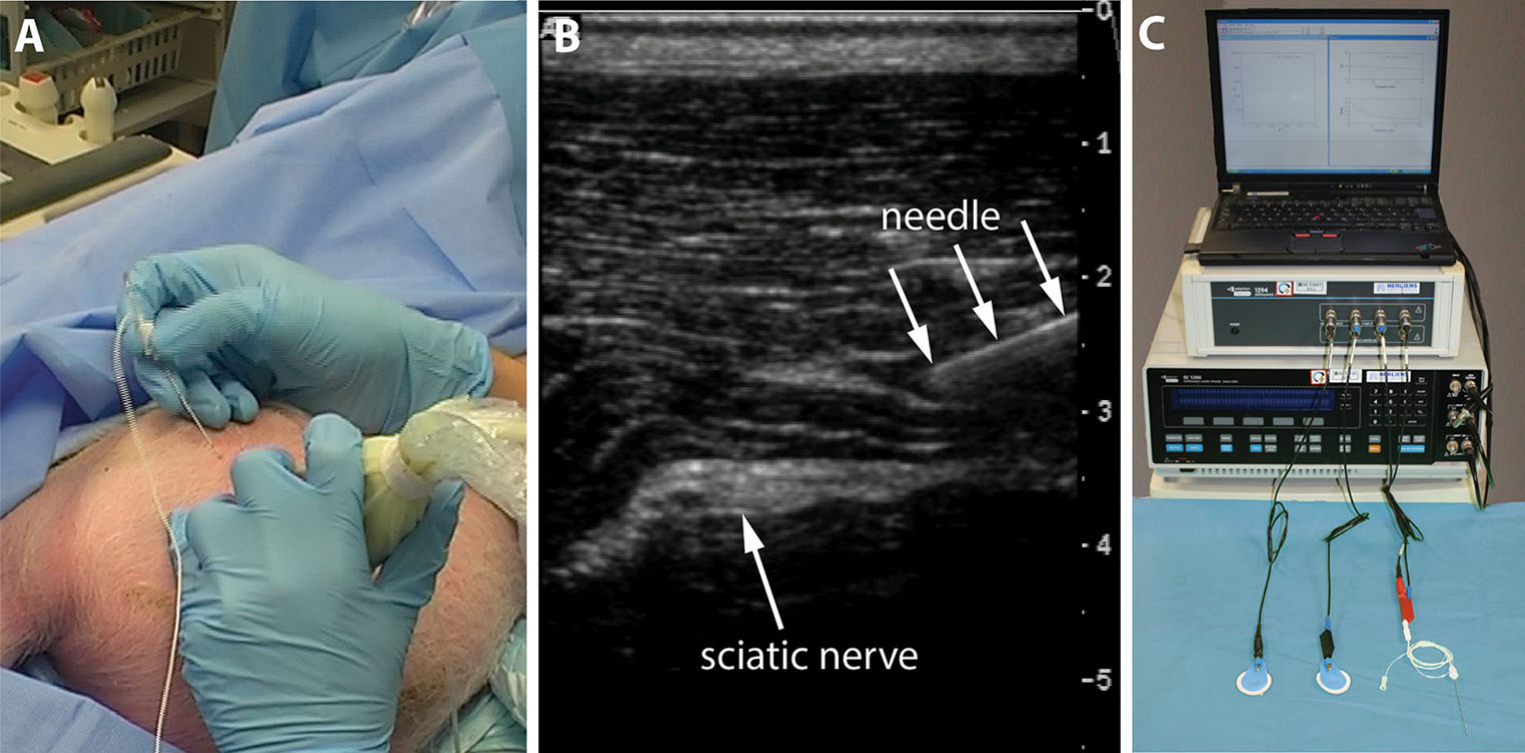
Study setup: The tip of a stimulation needle was placed under ultrasound guidance within the sciatic nerve and other tissue types (a and b). An impedance measurement system (Solartron 1260 and SI 1294) was connected for 3-electrode impedance measurements (c). Impedance as function of frequency was obtained by sweeping the excitation frequency in 25 logarithmically distributed steps from 1.26 to 398 kHz
